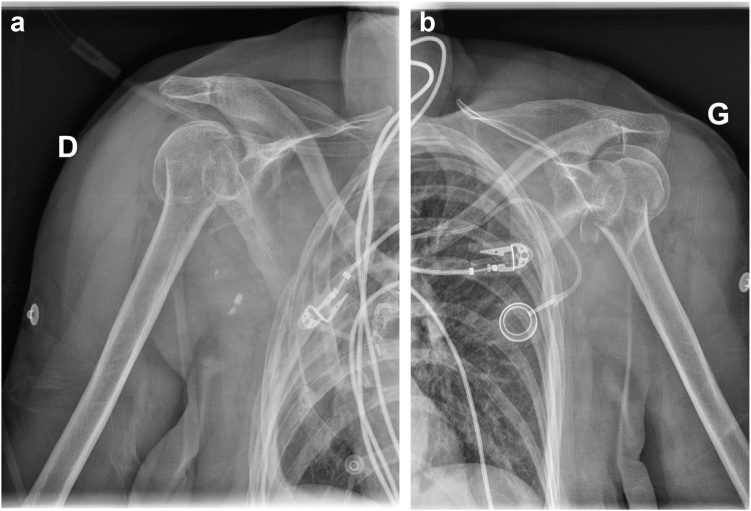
X-ray (anteroposterior) of Right (a) shoulder and Left (b) shoulder in Emergency, Department, showing bilateral posterior fracture-dislocation.
